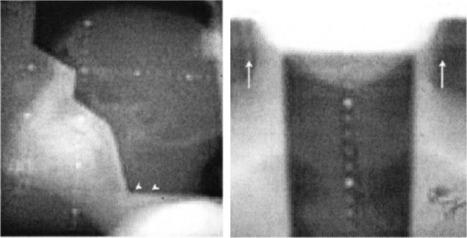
Clinical portal radiographs of a lateral cranial field and posterior (upper) spine field. Lead beads can be seen at the inferior field edge of the cranial portals and at the top level of the upper spine portal on the double exposure. The lead beads are taped to the immobilization mask at the level of $C_{2}$.
